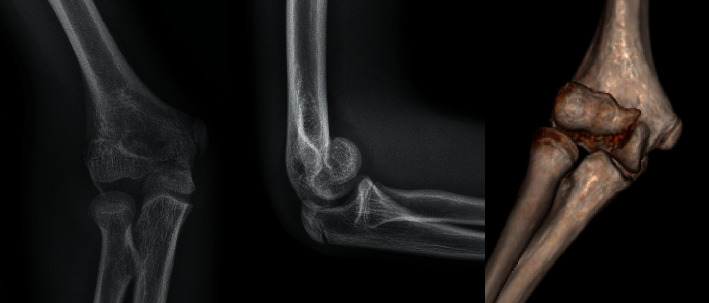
Patient number 4, preoperative X-rays, and CT scan. Type IV/McKee fracture according to Bryan and Morrey classification. Double arch sign is present on the lateral view.
